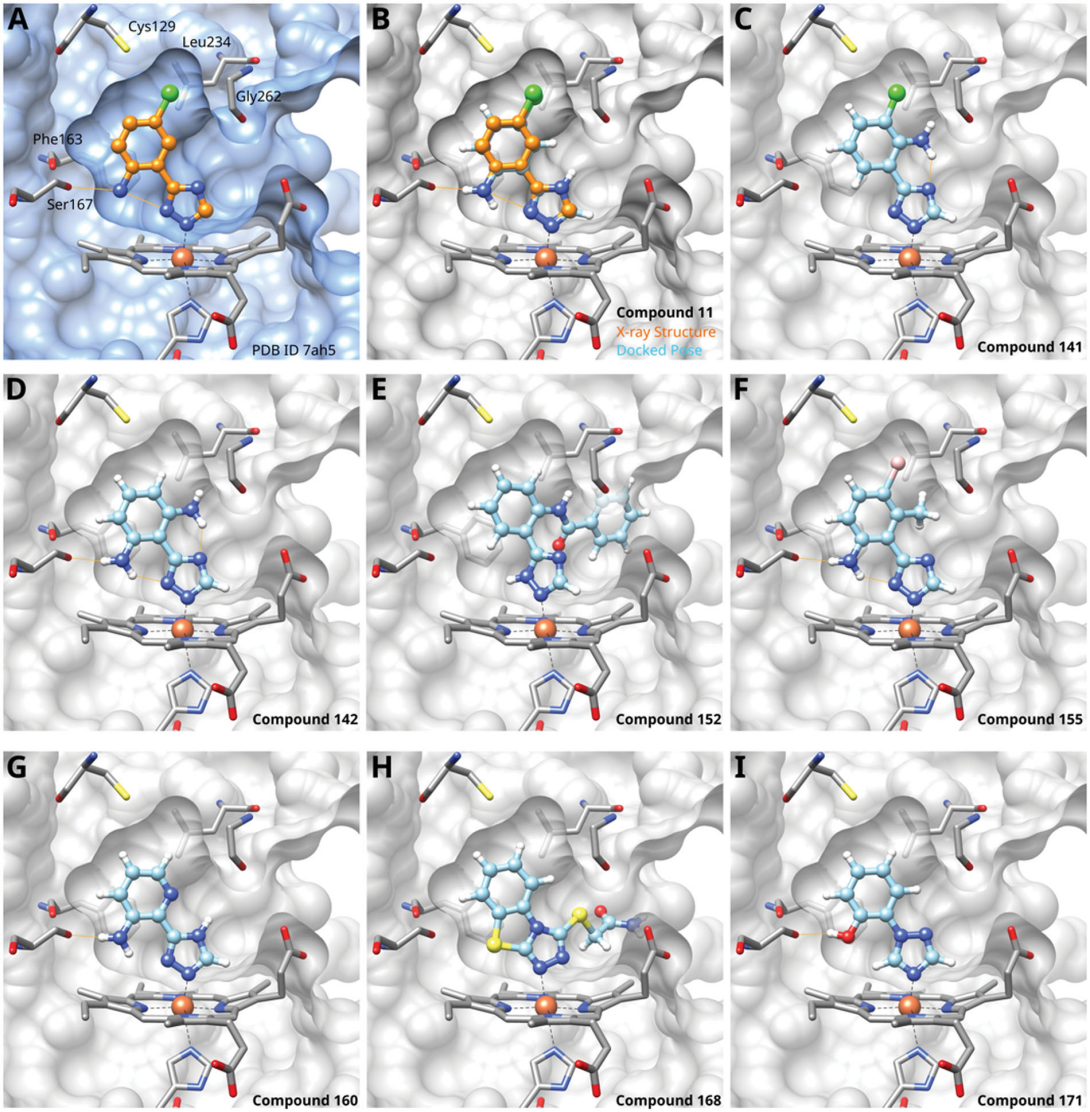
1,2,4-Triazoles. (A) X-ray structure of compound 11 (PDB ID 7ah5)^28^. (B) Superposition of X-ray structure and of docking pose of compound 11. Docking poses of (C) compound 141; (D) compound 142; (E) compound 152; (F) compound 155; (G) compound 160; (H) compound 168; (I) compound 171. Hydrogen bonds are displayed in orange.
